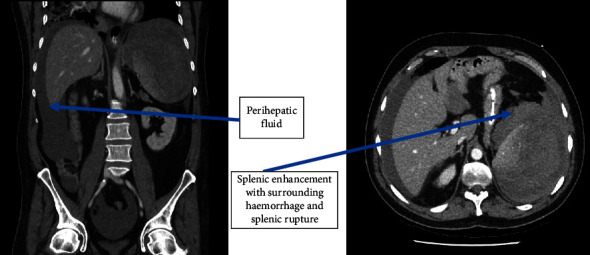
CT-abdomen and pelvis on day 3 of admission demonstrating large splenic laceration, haematoma, and haemoperitoneum.
